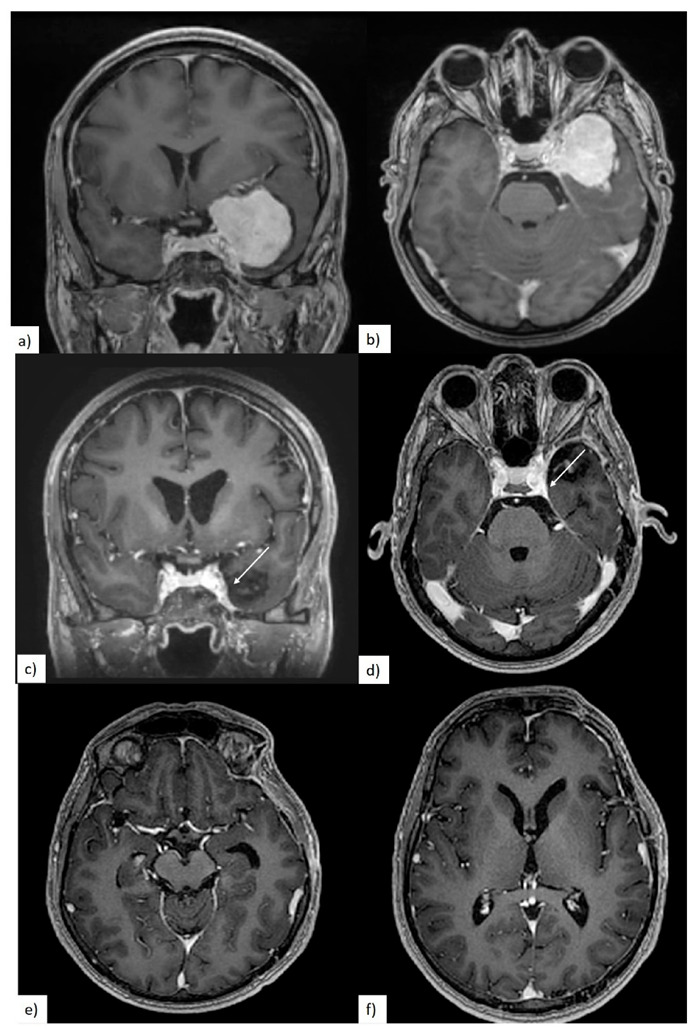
(a,b) Preoperative MRI showing cavernous sinus invasion; (c–f) postoperative MRI showing intracavernous remnant—white arrow.
